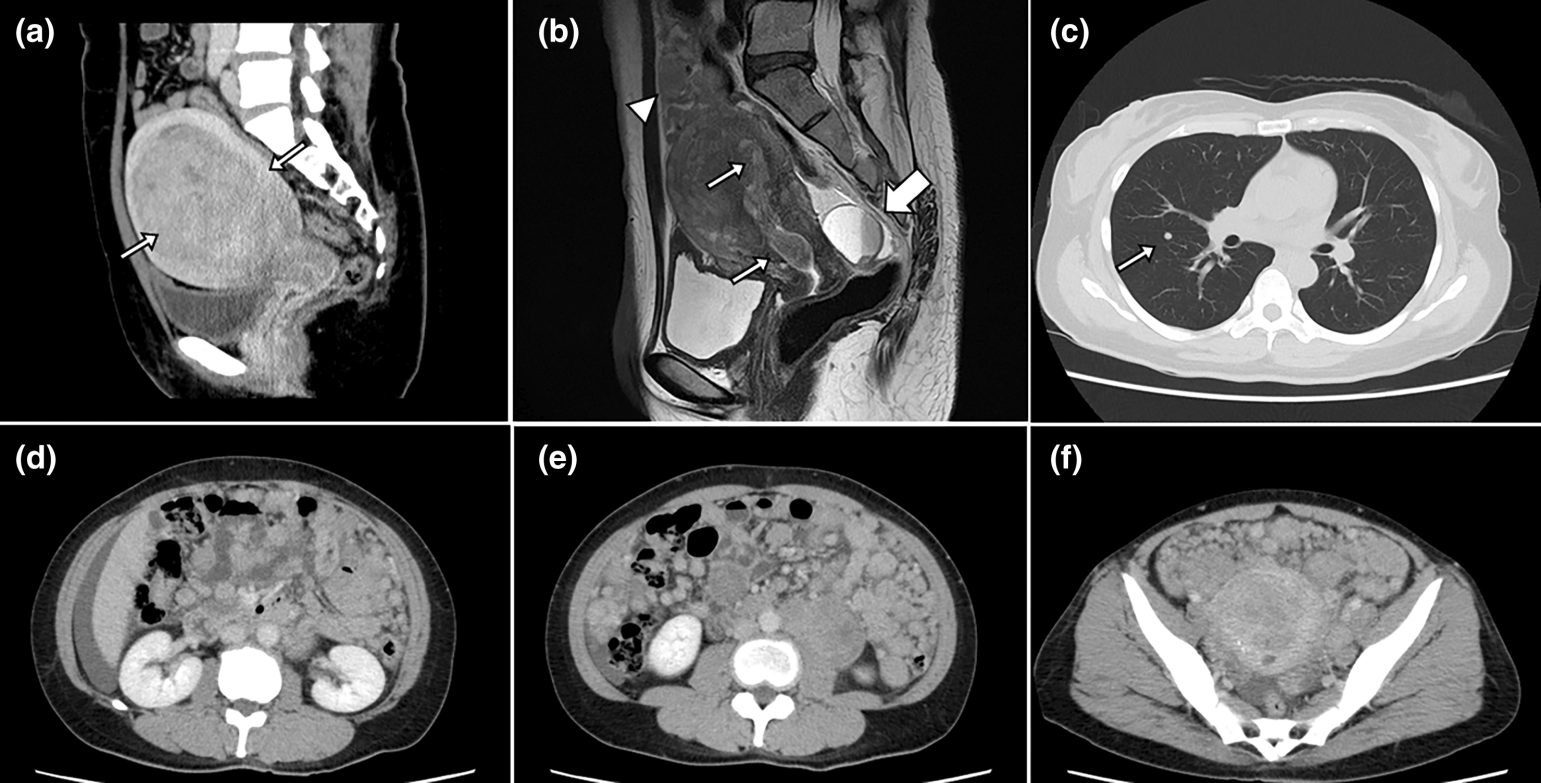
(a) Preoperative contrast‐enhanced computed tomography (CT) images from a laparoscopic myomectomy performed at a different hospital 6 years prior. One uterine myoma is found in the uterine body (arrows). (b) Preoperative T2‐weighted magnetic resonance images (MRI). The uterine body is enlarged, especially in the anterior wall, and many uterine myoma‐like mass lesions are observed in the uterine cavity and muscle layers (thin arrows). Furthermore, many nodular lesions (arrowheads) with contrast effects and mild diffusion restriction are seen in the abdominal cavity on contrast‐enhanced MRI (data not shown). A cystic lesion with a liquid surface formation is observed in the Douglas pouch, suspected to be an endometrial cyst or a peritoneal inclusion cyst (thick arrow). (c–f) Preoperative contrast‐enhanced CT images. Numerous nodular lesions of various sizes with contrast effects and clear borders throughout the entire abdominal area. A similar nodule is also observed in one location in the right lower lobe of the lung (arrow).
